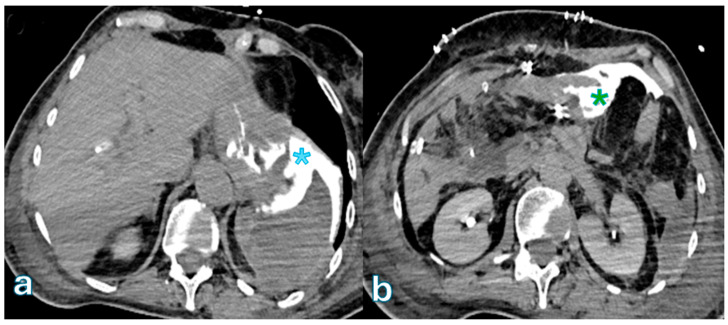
Axial CT with oral-administrated iodinated contrast showing the spread of extraluminal water-soluble contrast medium in a patient who underwent duodenopancreatectomy 3 days earlier. Oral contrast leakage is observed at the level of the gastrojejunostomy ((b) green asterisk), with distribution in the perisplenic region ((a), blue asterisk). These findings are indicative of gastrojejunostomy anastomosis dehiscence.
